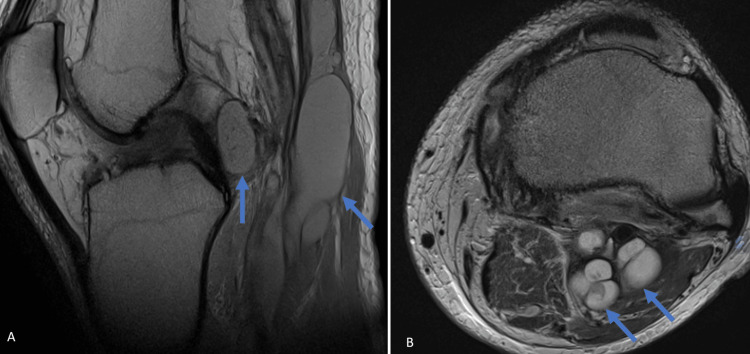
Preoperative T1-weighted non-contrast enhanced MRI of the left knee A: Preoperative T1-weighted non-contrast enhanced MRI of the left knee, sagittal scan, showing multiple iso-hyperintense well-circumscribed cystic lesions (blue arrows) in the popliteal fossa. B: Preoperative T1-weighted non-contrast enhanced MRI of the left knee, axial scan, showing two multilobulated, well-circumscribed hyperintense cystic lesions (blue arrows). Hyperintensity of the lesions is consistent with their mucinous components.
